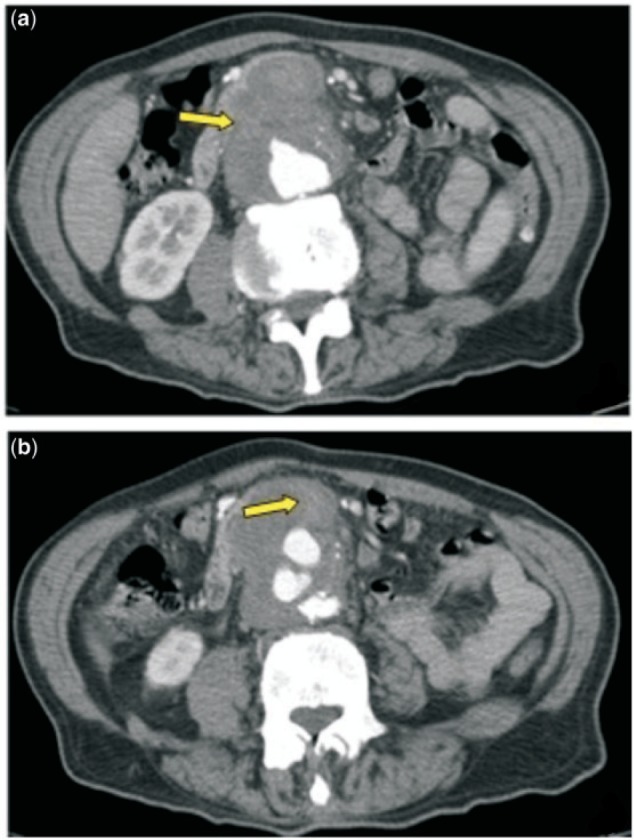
Enhanced CT scan; arrows showing: (a) absence of a clear separation between duodenal and aneurysmatic aortic wall; (b) fading contrast medium filling small bowel loop.
